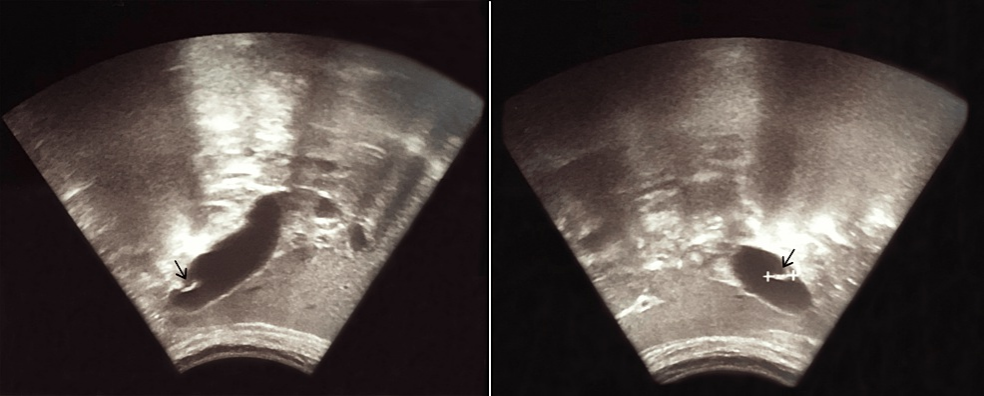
Abdominal ultrasound showing thickening of the gallbladder wall, with visualization of hyperechoic structures inside it, creating a tubular coiled appearance (arrow), without acoustic shadowing.
